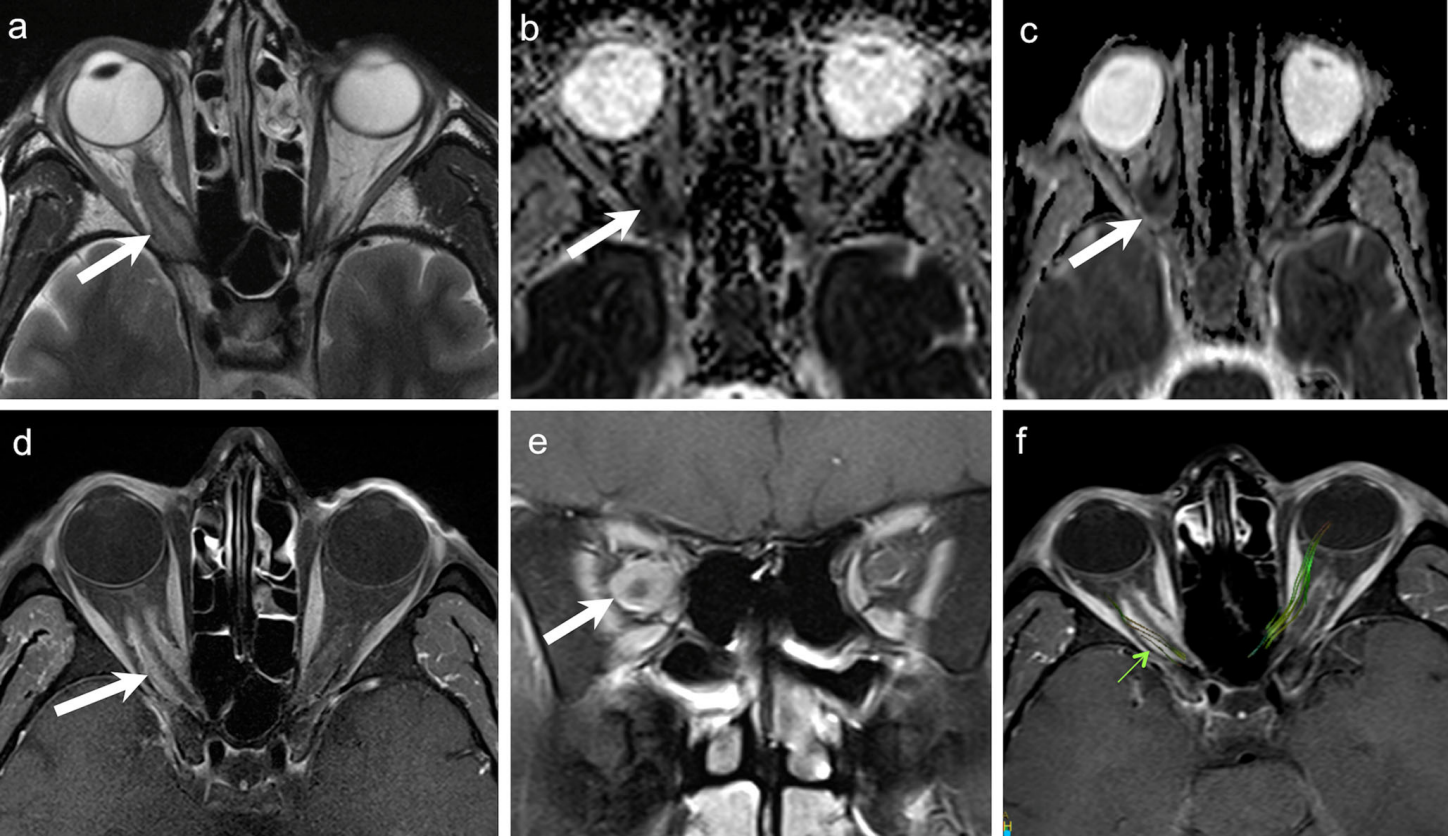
41-year-old woman with right-sided progressive vision loss and proptosis. a. Axial T2W image shows a moderately hpointense fusiform lesion originating from the optic nerve sheath encasing the optic nerve (arrow). b. Corresponding axial ADC map from RESOLVE DWI shows restricted diffusion (arrow). Note only minor image deformation. c. ADC map from standard EPI sequence also shows restricted lesion diffusivity (arrow); however, note major image deformation due to susceptibility artefacts. d. Axial contrast enhanced T1W image reveals strong fusiform enhancement along the optic nerve (“tram track sign”, arrow). There was no extension through the orbital apex intracranially. e. Coronal fat saturated T1W image displaying concentric enhancement of the tumour around the compressed optic nerve creating a characteristic “bull’s eye” appearance (arrow). Imaging findings are in keeping with an ONSM. f. DTI 3D tractography reconstruction of the optic nerves reveals normal fibres on the left (fibers are depicted in green due to their anterior- posterior course) and major fibre atrophy on the right (arrow)
